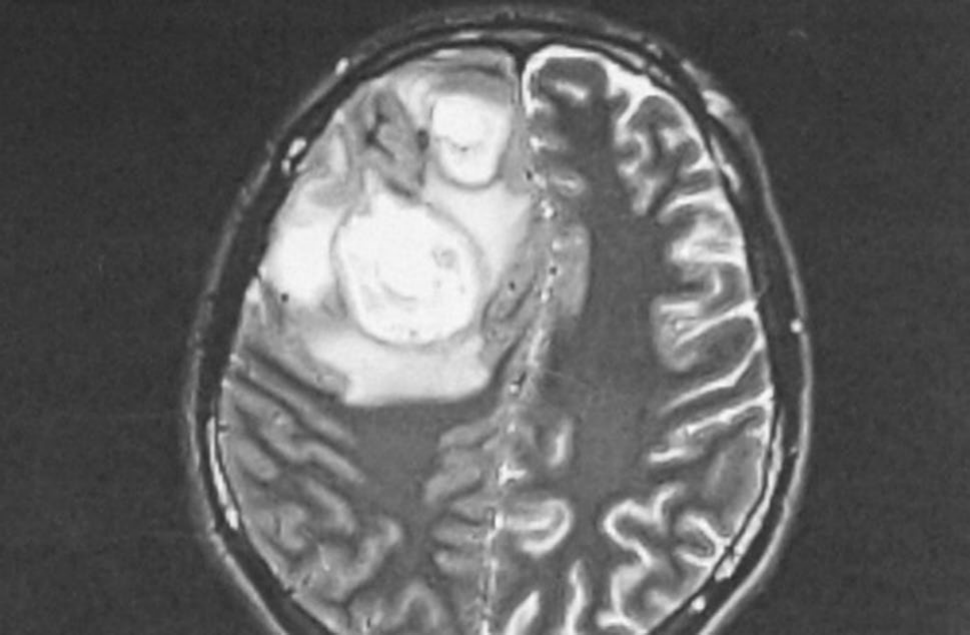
MRI of the cranium in a 28-year-old man. Two abscesses with diameters of 45 and 28 mm in the right frontal lobe. There is oedema of the brain tissue and thickened cerebrospinal fluid over the area where the abscess is located. The mass is clearly visualised and compression and displacement of the right chamber are also noted
